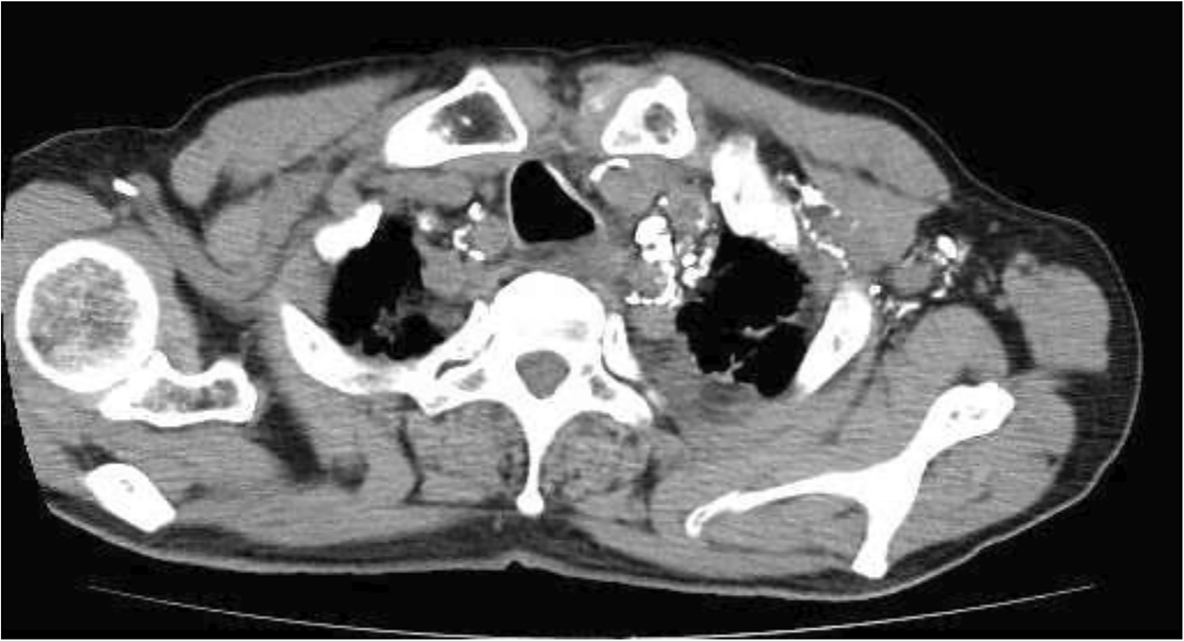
Computed tomography image showing enhanced supraclavicular lymphatic vessels
